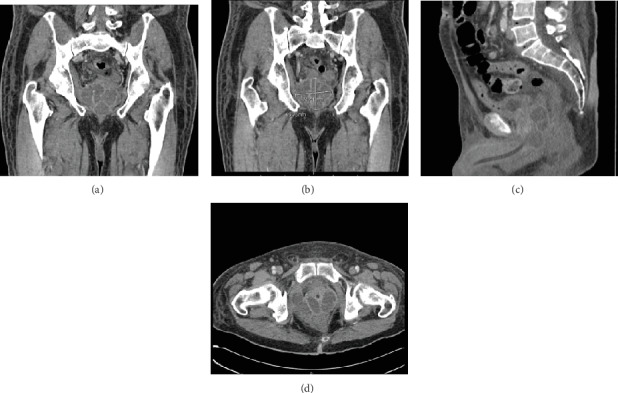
CT of the pelvis with contrast showing the multiloculated prostatic abscess in: (a) coronal view, (b) coronal section with measurements, (c) sagittal view showing involvement of the right perirectal/perianal region, and (d) axial view showing invasion of the right pelvic sidewall/obturator muscle.
